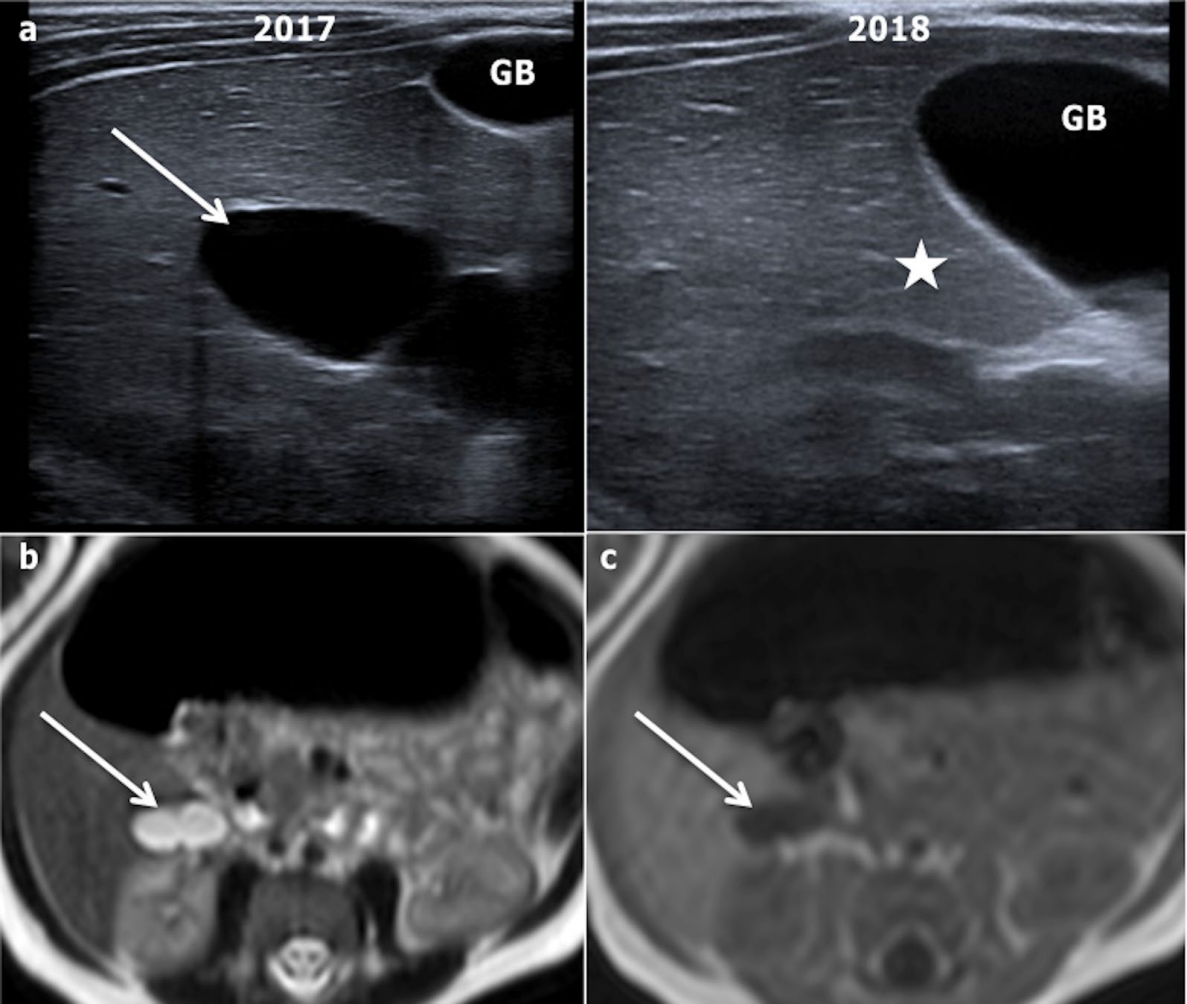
Spontaneous disappearance of a cystic lesion in a 5-year-old boy between 2017 and 2018, which led to the diagnosis of this lesion as a most likely liver lymphatic malformation. a Ultrasonography shows biloculated homogeneous cyst (arrow), which vanished one year later (star). It displayed fluid signal (arrows) on axial T2-weighted (b) and T1-weighted magnetic resonance imaging in 2017 (c). The main differential diagnosis of this lesion would be two fused simple hepatic cysts or mesenchymal hamartoma
